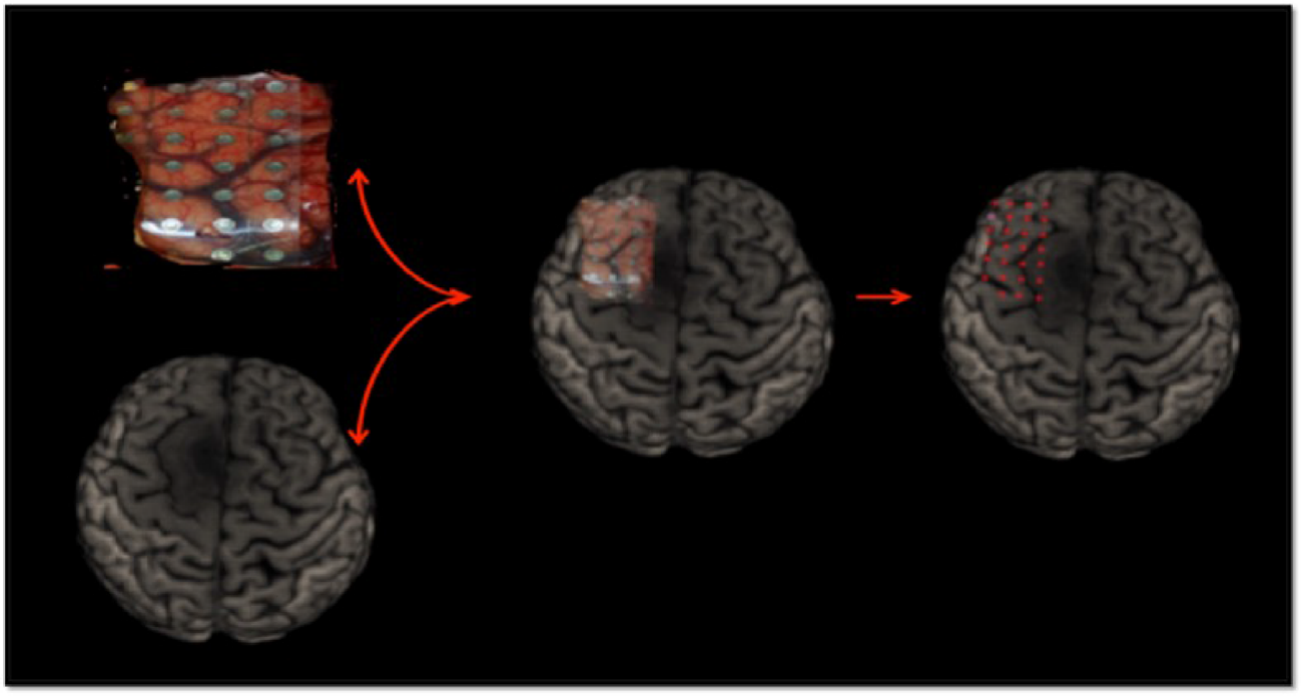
ECoG localization method *via* intra-operative photograph merged with the cortical surface segmented from the pre-operative MRI.
